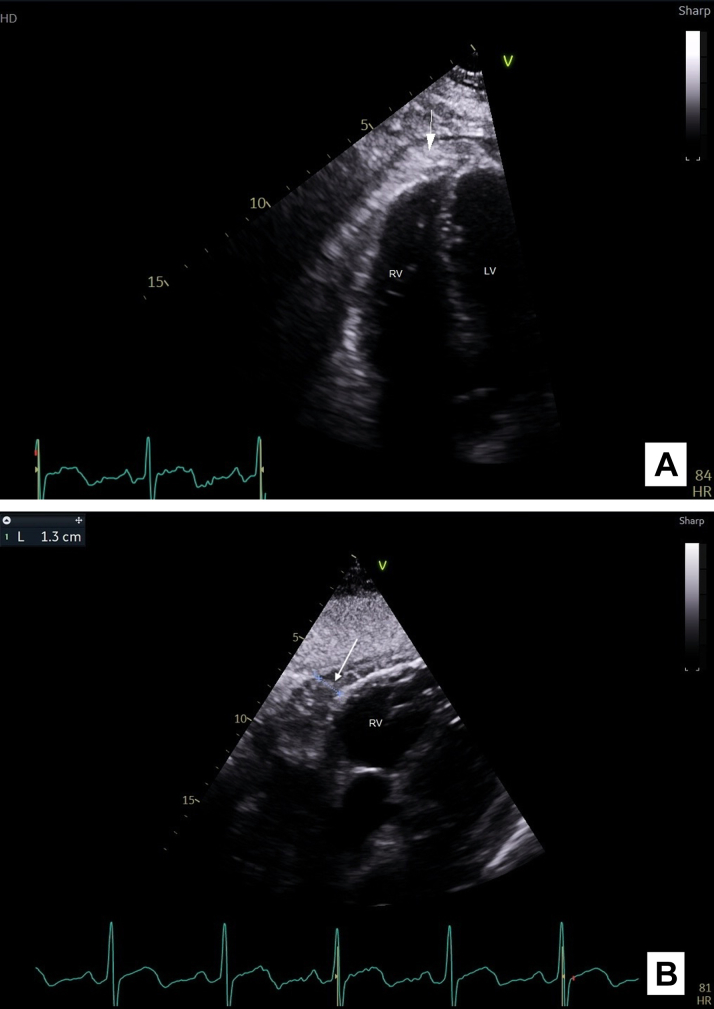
Repeat Transthoracic Echocardiogram Transthoracic echocardiogram (TTE) performed 24 hours following the initial TTE (day 17 of admission), apical 4-chamber view (A) and subcostal view (B) showing dense echogenic material in the pericardium (white arrows) adjacent to the right ventricle, increased in size compared to previous image. LV = left ventricle; RV = right ventricle; TTE = transthoracic echocardiogram.
